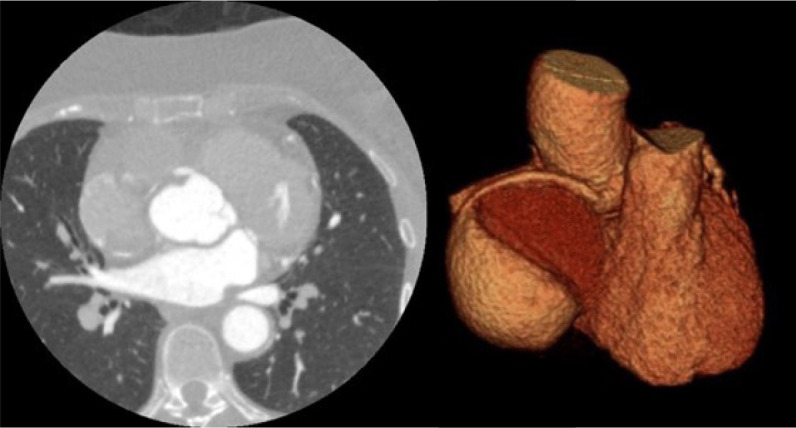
A) Coronary computed tomography angiography showing a saccular aneurysm with a partially calcified mural thrombus. B) Three-dimensional reconstruction confirming that the mass was supplied by the right coronary artery.
